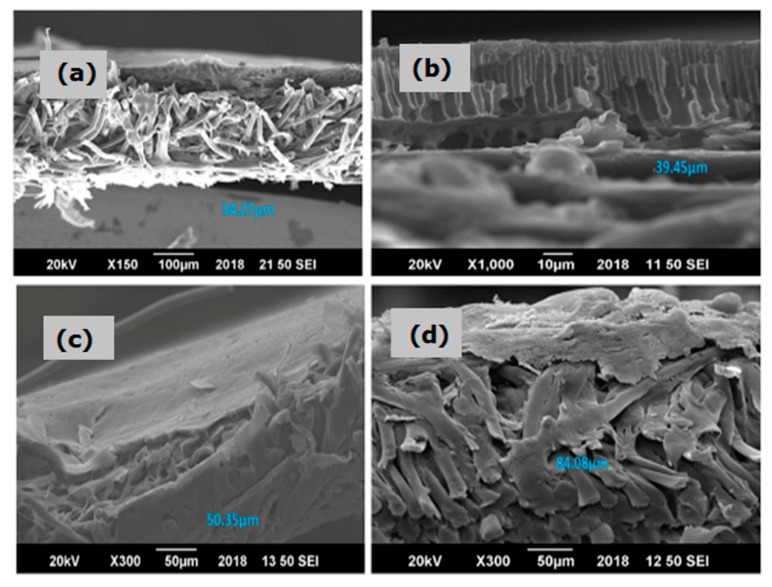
SEM image showing the morphology and width of the (a) pure polyethersulfone (PES) membrane, (b) cannabinoid embedded polyethersulfone (C-PES) membrane, (c) terpene embedded polyethersulfone (T-PES) membrane, and (d) cannabinoid and terpene embedded polyethersulfone (CT-PES) membrane.
